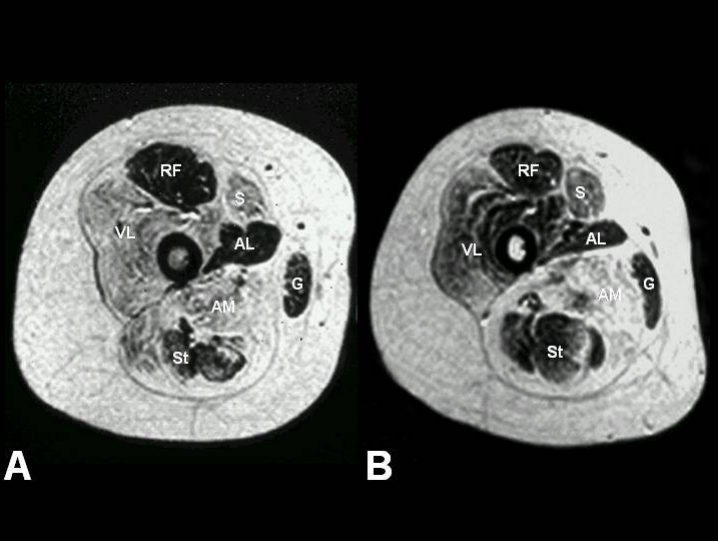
Selective muscle involvement in *RYR1*-related central core disease (CCD) and multi-minicore disease (MmD). Muscle MRI of the thigh, T1-weighted images. A) A transverse section from the proximal thigh in a 12-year-old patient with central core disease (CCD) due to a dominant mutation in the skeletal muscle ryanodine receptor *(RYR1) *gene. There is a distinct pattern of selective involvement characterized by marked increase in signal within the vasti, sartorius (S), and adductor magnus (AM), and relative sparing of the rectus femoris (RF), adductor longus (AL), gracilis (G), and hamstring muscles. B) A transverse section from the proximal thigh in a 17-year-old girl with Multi-minicore disease due to a homozygous recessive *RYR1 *mutation demonstrating a comparable pattern of selective involvement. * Reprinted from [24] Neurology 2002 Jul 23;59(2):284–7. Jungbluth *et al*.: Autosomal-recessive inheritance of *RYR1 *mutations in a congenital myopathy with cores.With permission from Lippincott Williams & Wilkins (LWW).
